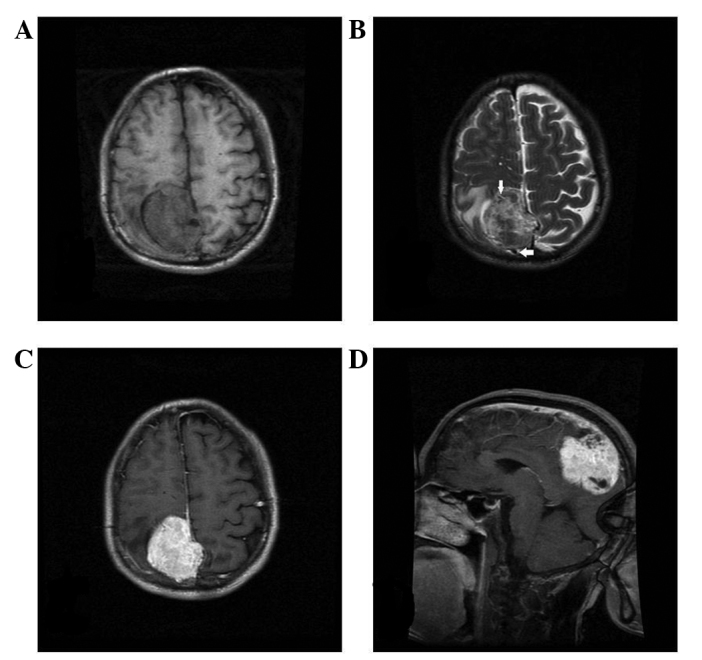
Patient 1. (A) Unenhanced magnetic resonance imaging scan reveals a heterogeneous iso-signal intensity mass in the right occipital region with a well-defined border. (B) The lesion has a marginally long T2 signal with certain intratumoral vessels (arrow), and peritumoral brain edema may be observed. (C) Enhanced scan demonstrates that the mass was markedly heterogeneously enhanced. (D) Cystic degeneration, necrosis and flow void are visible.
